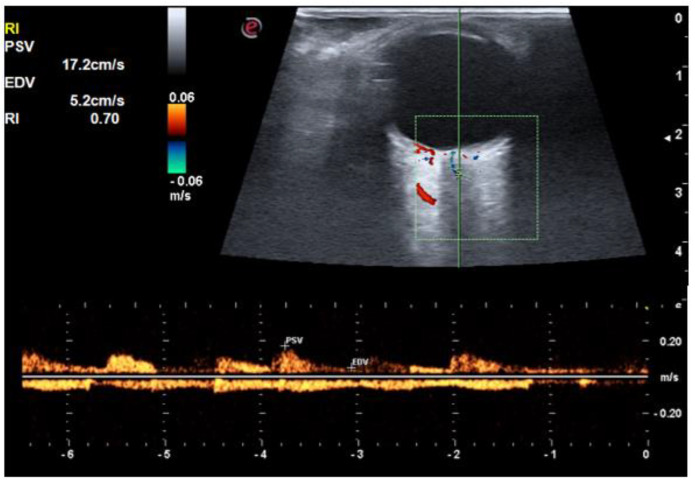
A color Doppler image of a diabetic patient without retinopathy shows a resistive index of 0.70 in central retinal artery.
